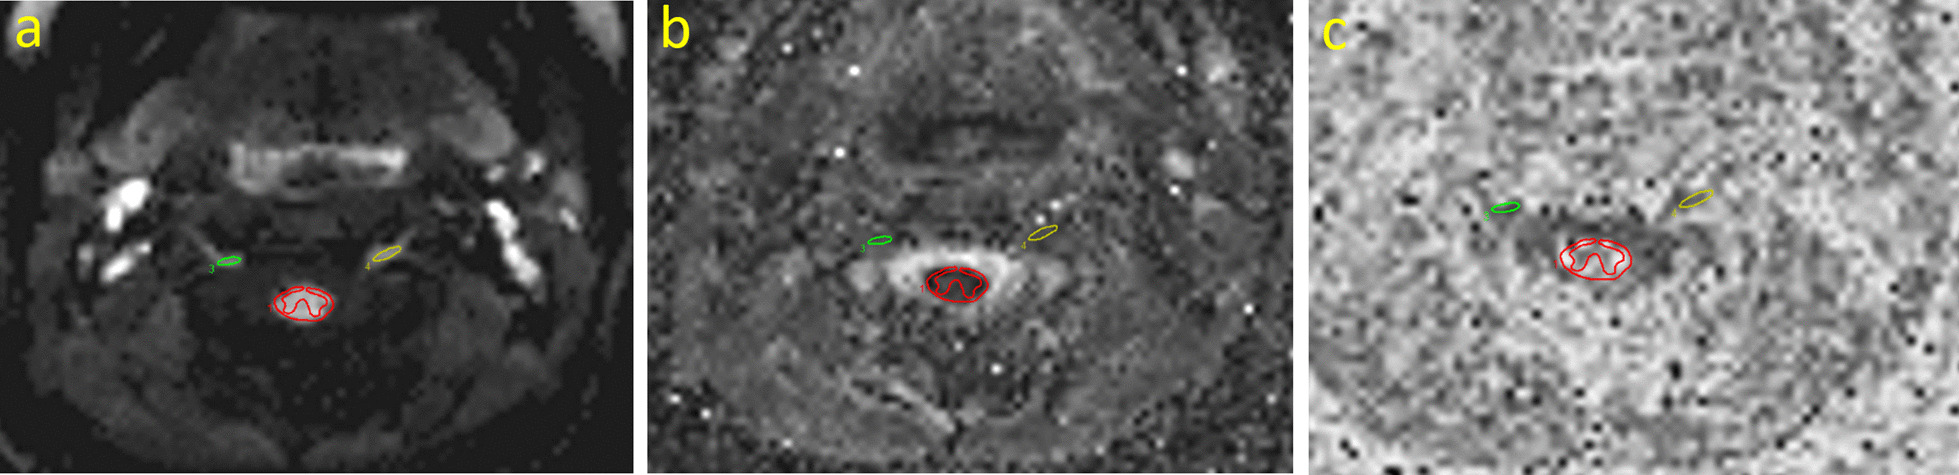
Diffusion MRI examples. *Legend*
